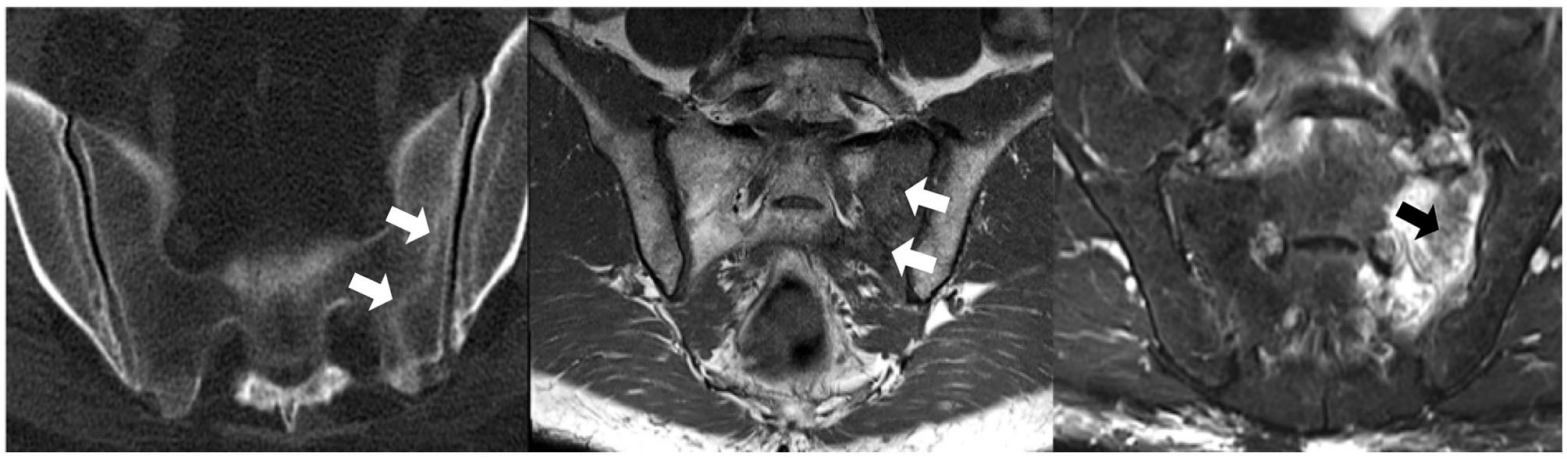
CT (left), T1WI (middle), and fat-saturated PD (right) MRI of a patient with sacral insufficiency fractures (arrows). T1WI shows diffuse slightly hypointense signal of the left sacral wing and a linear hypointensity compatible with a sacral fracture; corresponding fat-saturated PD image documents marked bone marrow edema.
